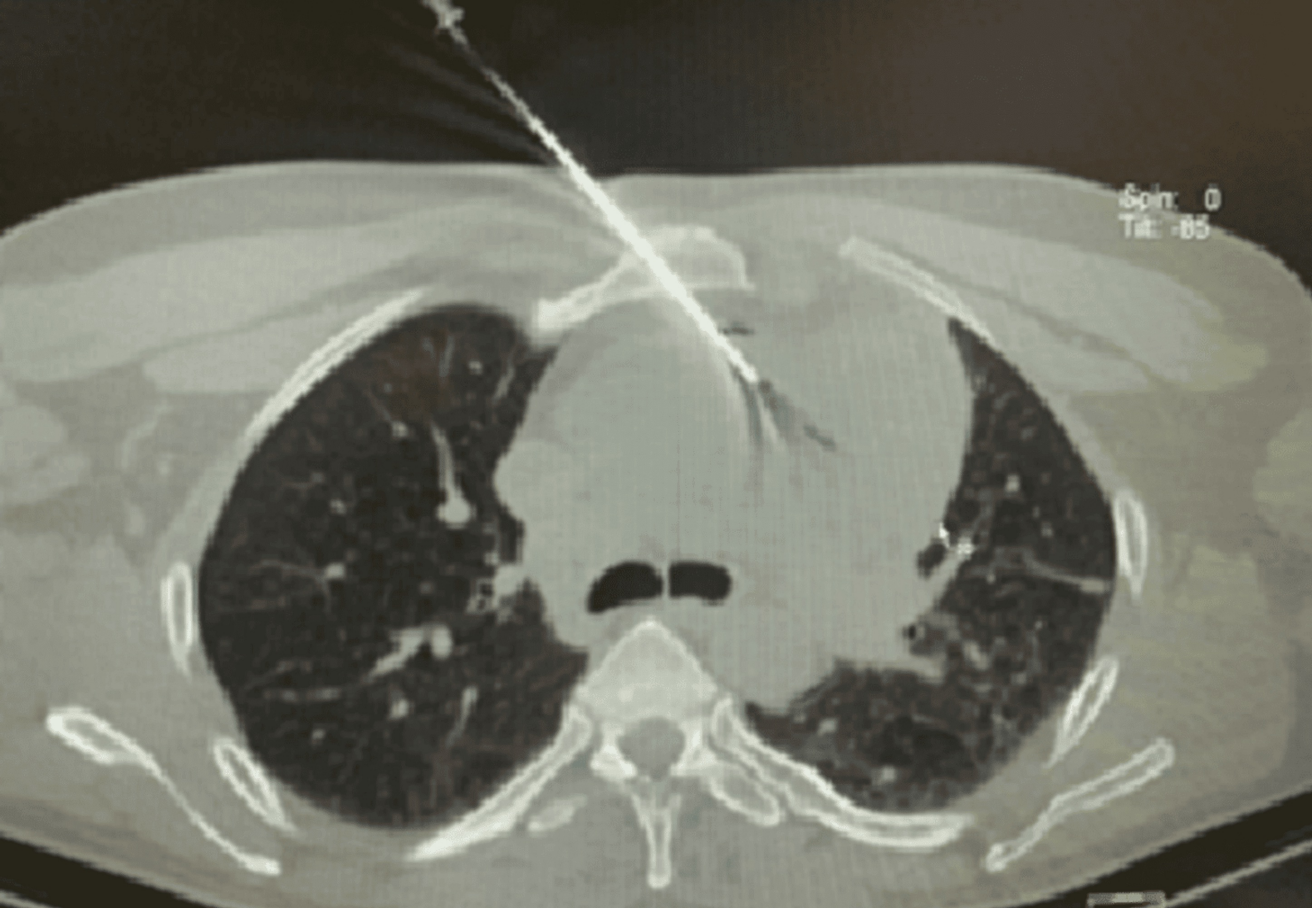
Computed tomography (CT)-guided transsternal biopsy of mediastinal mass
